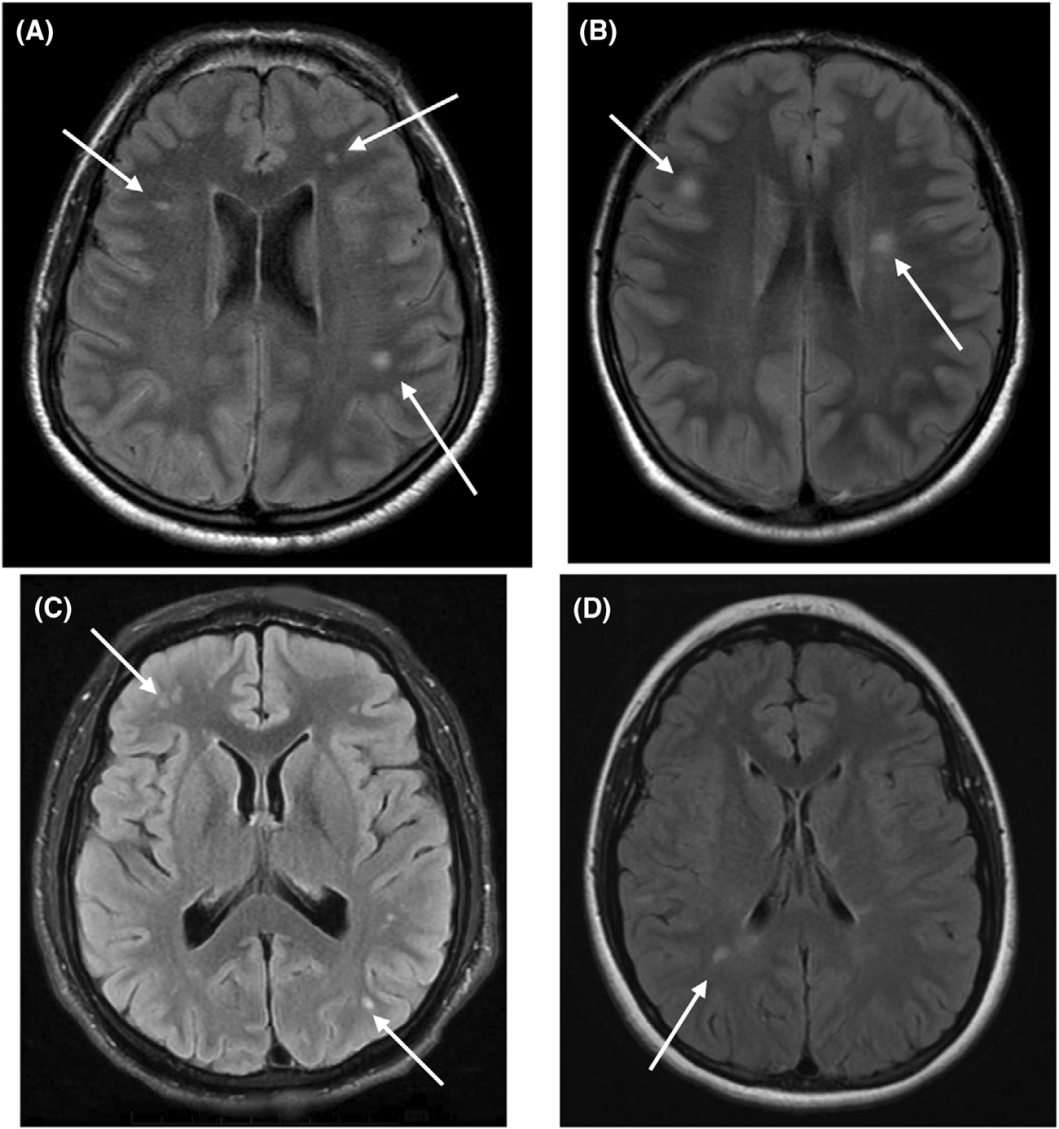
Examples of T2/FLAIR signal abnormalities in individuals DSRD. (A–D) Axial T2/FLAIR sequences demonstrating focal T2 signal prolongation throughout the white matter. White arrows highlight characteristic lesions identified in the white matter.
